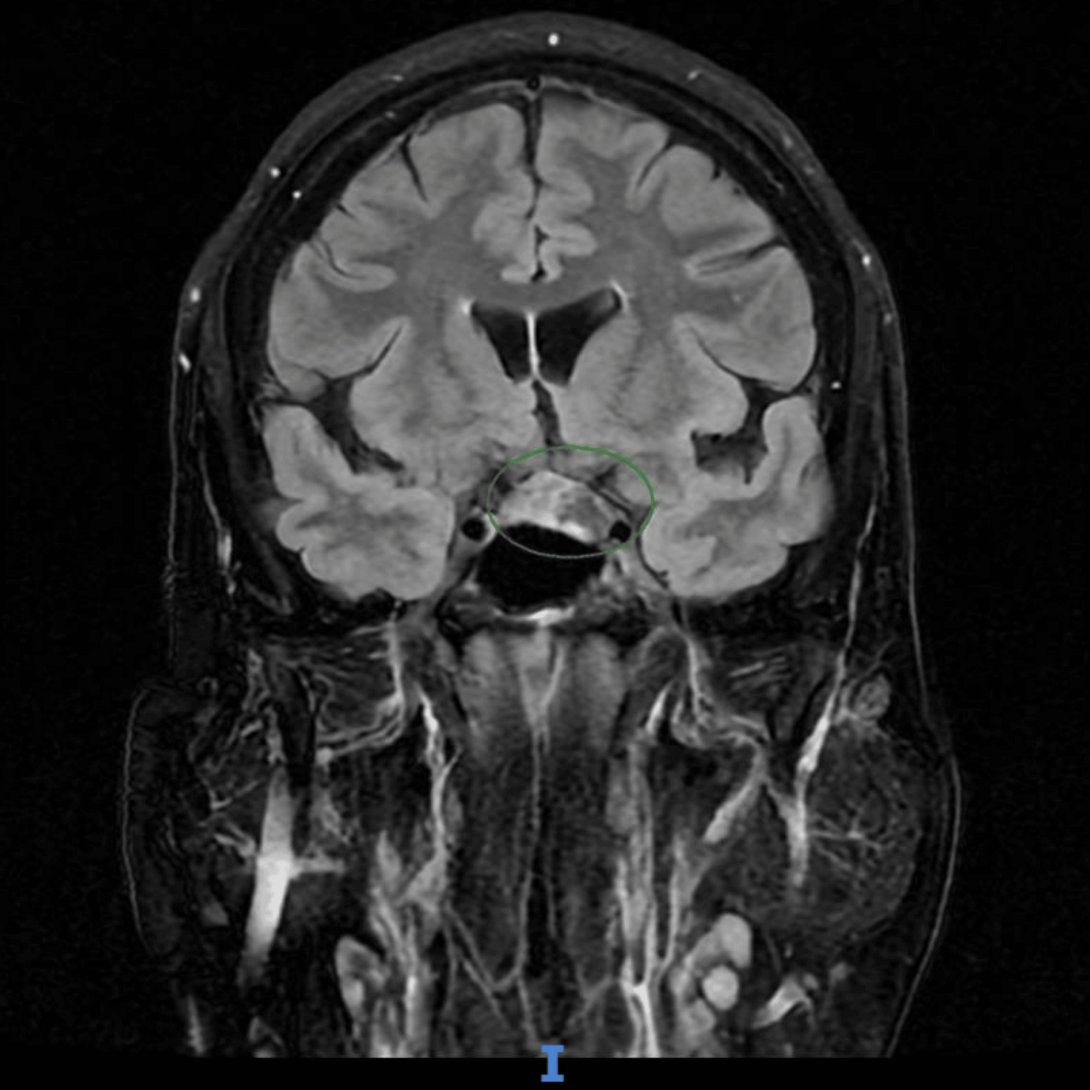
MRI of the head. There was a bulky/enlarged left pituitary gland, with high T1-weighted (T1W) signal, and corresponding low T2 signal, in the left lobe, which may represent hemorrhagic changes. A 0.4 cm non-specific focus of high T2-weighted (T2W)/flare signal in the left cerebral hemisphere - corona radiata was noted. A single focus of susceptibility artefact in the left per occipital deep white matter on susceptibility weighted imaging was present. Otherwise, the normal appearance of the brain parenchyma and ventricles was present, with no acute infarct.
